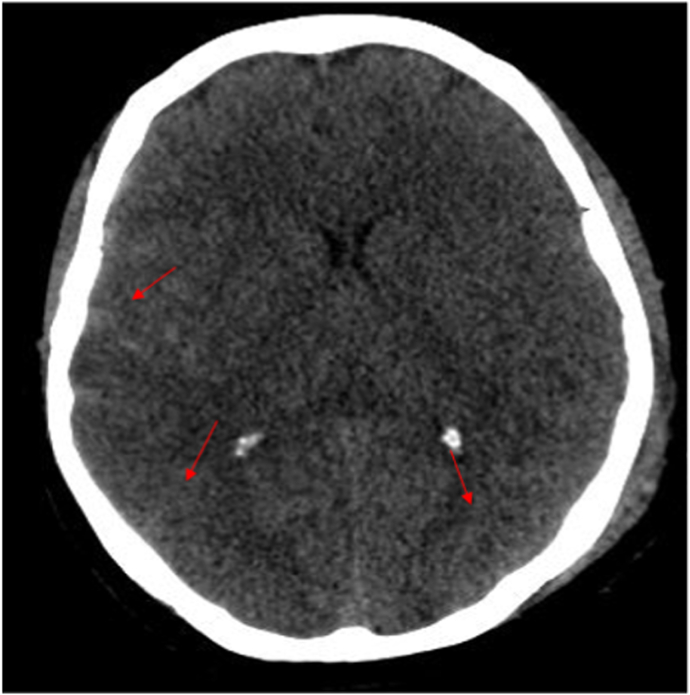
Axial view of a cerebral CT scan revealing A: a moderate meningeal hemorrhage B: hemorrhagic contusion in the parietal, basifrontal, and temporal areas.
